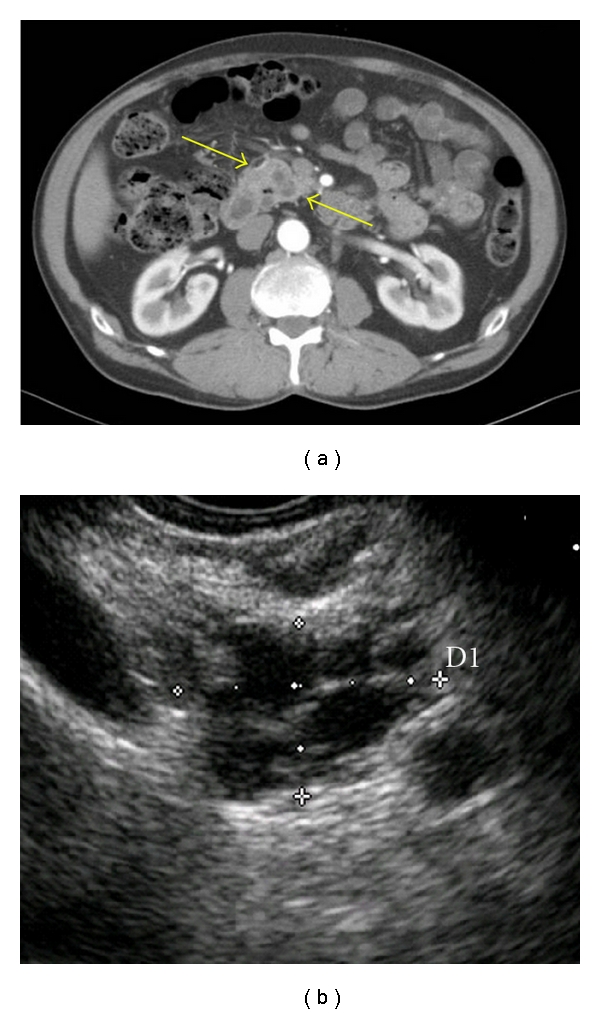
Main-duct intraductal papillary mucinous neoplasm. (a) Typical CT (arrows) and (b) EUS (cross marks) appearance.
